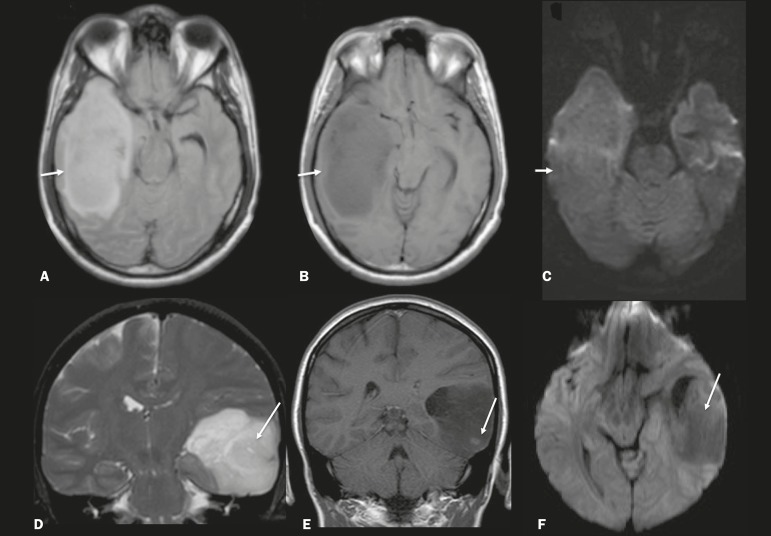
MRI of glioma. A: FLAIR sequence showing a hyperintense signal in the cortico-subcortical portion of the right temporal lobe. B: Axial T1-weighted sequence showing a hypointense signal with an expansile effect in the right temporal lobe. C: DWI showing no restricted diffusion. (A, B, and C all represent a patient with low-grade astrocytoma.) D: Coronal T2-weighted sequence showing an expansile lesion, with cortical thickening, in the temporal lobe. E: Coronal, contrast-enhanced T1-weighted sequence with a focus of nodular impregnation. F: DWI showing no restricted diffusion. (D, E, and F all represent a patient with ganglioglioma.)
